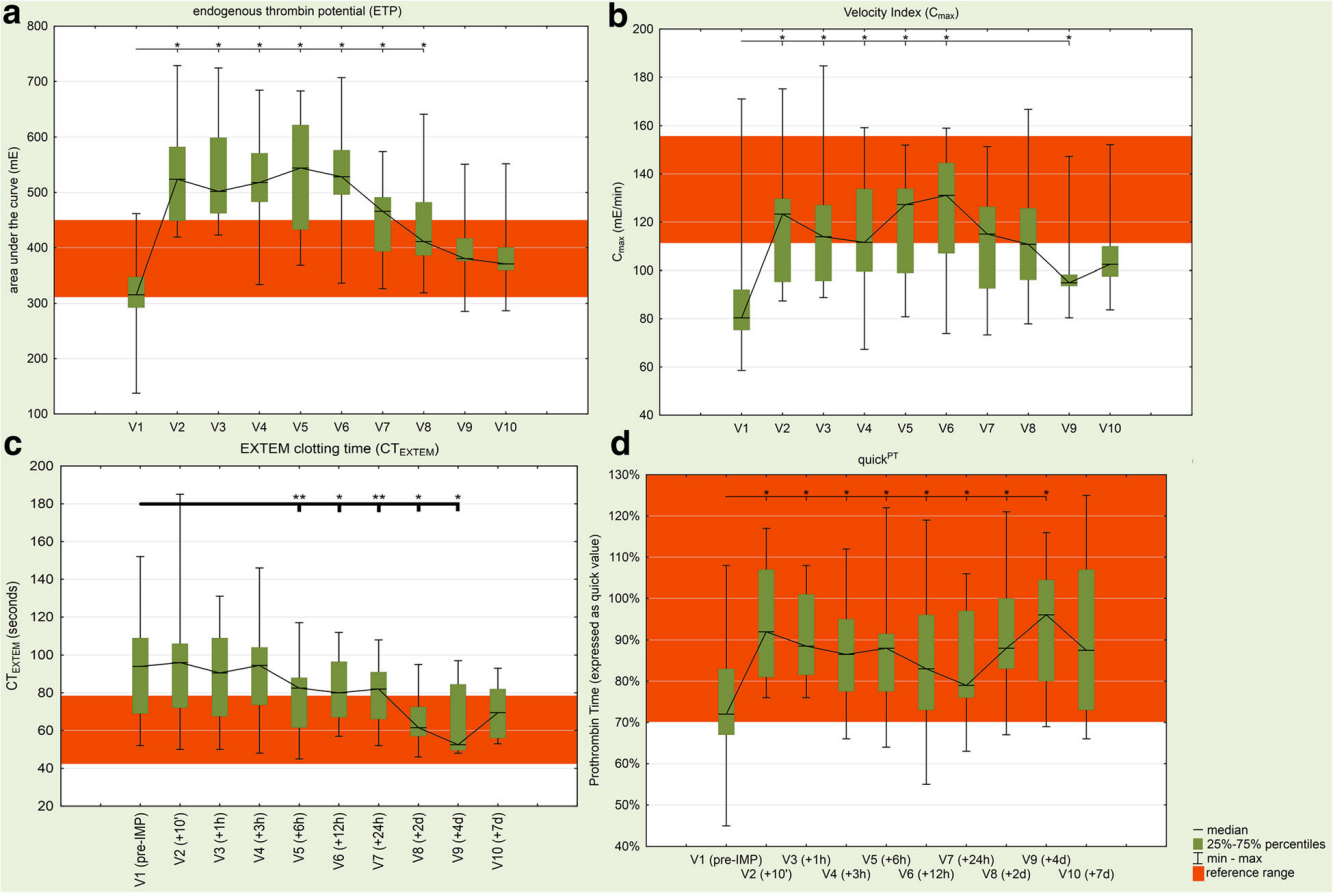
Effect of four-factor PCC on coagulation parameters. ETP (a), C_max_ (b), CT_EXTEM_ (c) and Quick^PT^ (d) for all patients from V1 to V10. Normal (healthy persons without anticoagulation) values are indicated (red area). Significant differences from baseline were determined using the Wilcoxon signed-rank test (p* < 0.05, *p* < 0.01). Abbreviations: ETP, endogenous thrombin potential; C_max_, thrombin generation, peak thrombin generation; CT, ROTEM®, clotting time; EXTEM, ROTEM®, extrinsic coagulation pathway; Quick^PT^, Quick value (prothrombin time)
